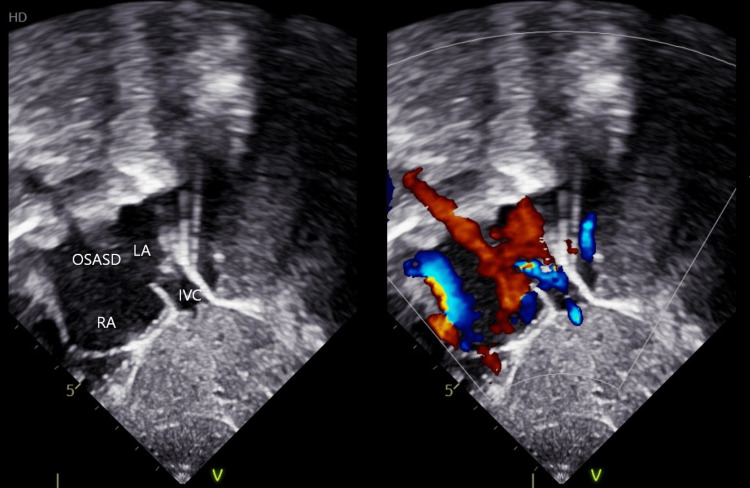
Case 2 - Echocardiography showing IVC draining into the LA. IVC: inferior vena cava: LA: left atrium, RA: right atrium
